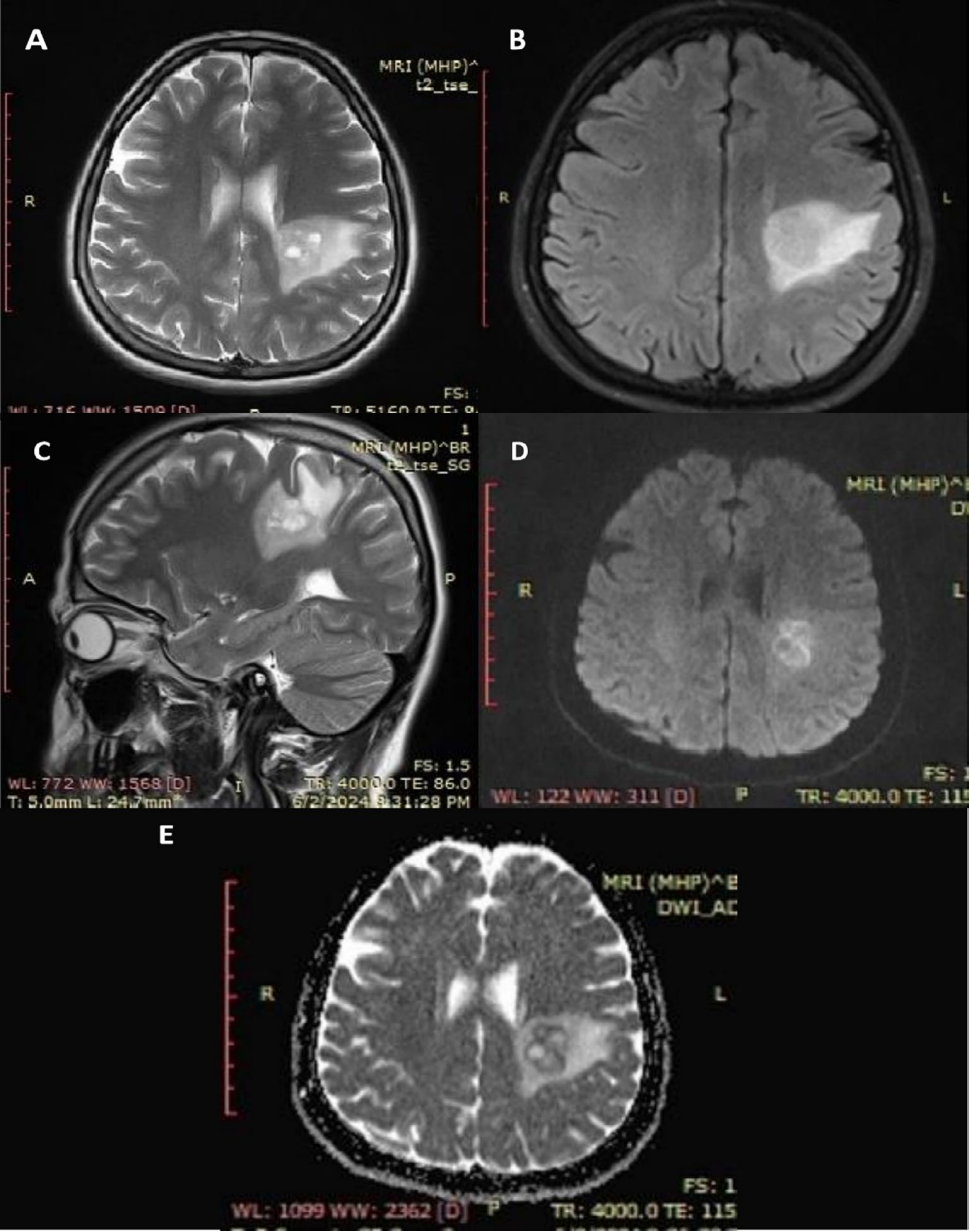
First brain MRI 2 months after initial presentation. (A) T2 Axial, (B) FLAIR Axial, (C) T2 Sagittal, (D) DWI, (E) ADC Map. T2 axial, sagittal and flair axial reveal left (LT) fronto‐parietal cystic mass surrounded by mild vasogenic edema. DWI and ADC images reveal diffusion restriction of the wall and the septa of the cystic mass.
